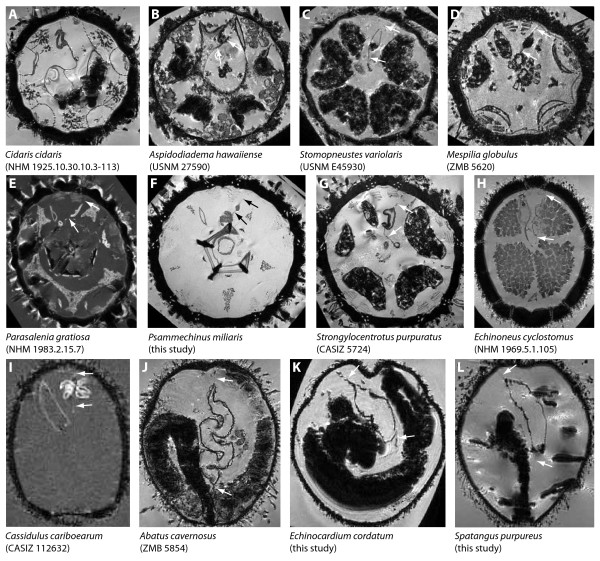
Homology of the sea urchin gastric caecum based on its integration into the mesenterial system, in particularl the dorso-ventral mesentery, as a primary criterion. Virtual horizontal sections based on MRI scans of Cidaridae (A), Aspidodiadematidae (B), Stomopneustidae (C), Temnopleuridae (D), Parasaleniidae (E), Parechinidae (F), Strongylocentrotidae (G), Echinoneidae (H), Cassidulidae (I), Schizasteridae (J), Loveniidae (K), and Spatangidae (L). The gastric caecum - where present - is attached to esophagus, axial complex, and the test through the dorso-ventral mesentery (arrows). In the Cidaroida (A), the dorso-ventral mesentery attaches to the single festoon present in ambulacrum III. In the more derived spatangoid and certain holasteroid taxa, this mesentery is shifted away from its original insertion near ambulacrum III towards interambulacrum 3, resulting in an oblique position of the gastric caecum (K, L). Note that the gastric caecum, in contrast to the rest of the digestive tract, is always free of sediment grains in the burrowing irregular taxa (H-L). Not to scale.
